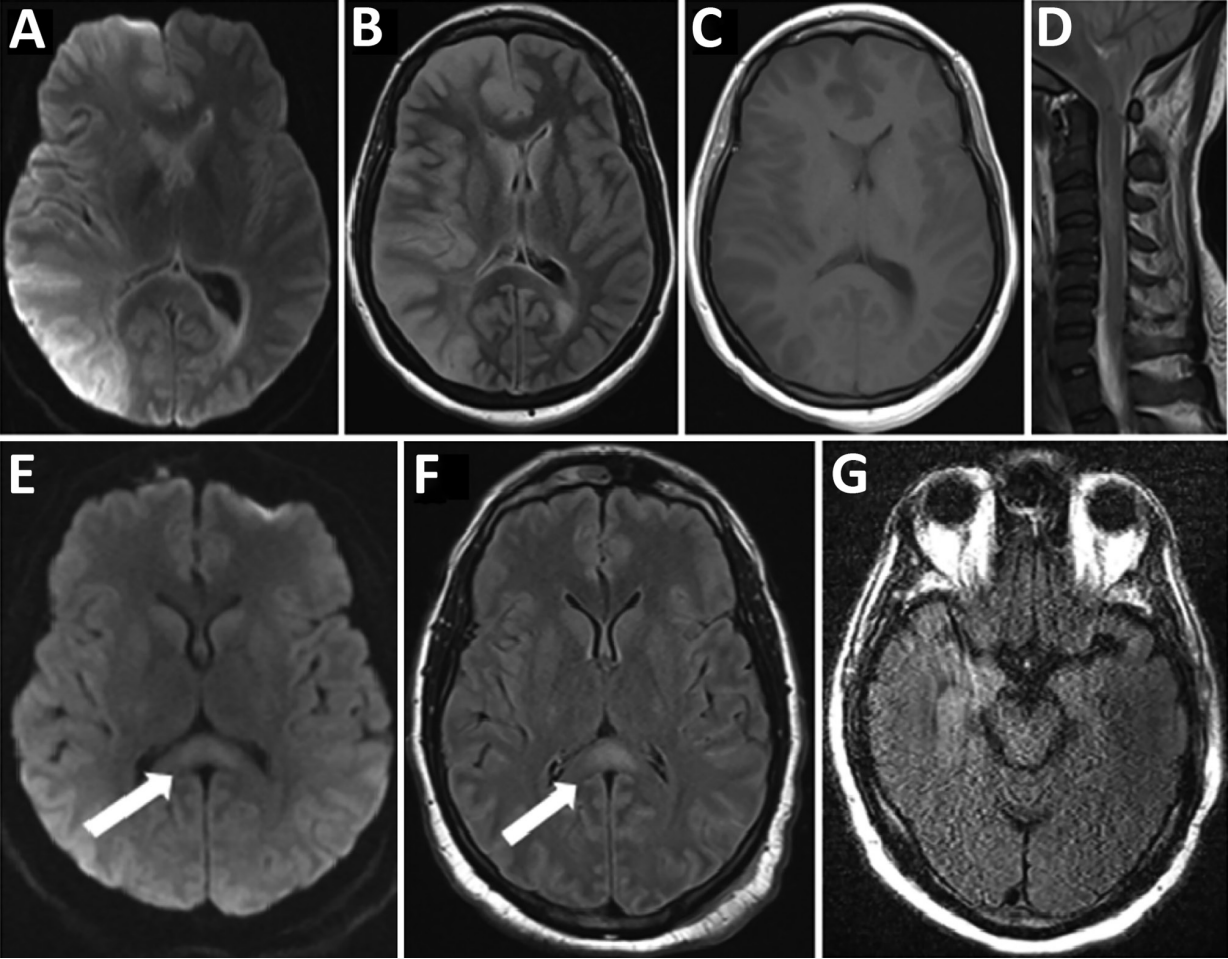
Magnetic resonance imaging findings for 3 patients with coronavirus disease who had neurologic complications, Atlanta, Georgia, USA, 2020. A–D) Patient 1 had right cerebral hemispheric restricted diffusion (diffusion weighted imaging in panel A) and cerebral edema (fluid-attenuated inversion recovery [FLAIR] in panel B) affecting gray matter and deep gray nuclei, without enhancement (panel C), and spinal edema (panel D). E, F) Patient 2 had a splenium lesion (diffusion weighted imaging in panel E and FLAIR recovery in panel F that was nonenhancing). Arrows indicate lesions in the splenium. G) Patient 3 had an equivocal fluid-attenuated inversion recovery FLAIR abnormality in the right temporal lobe.
